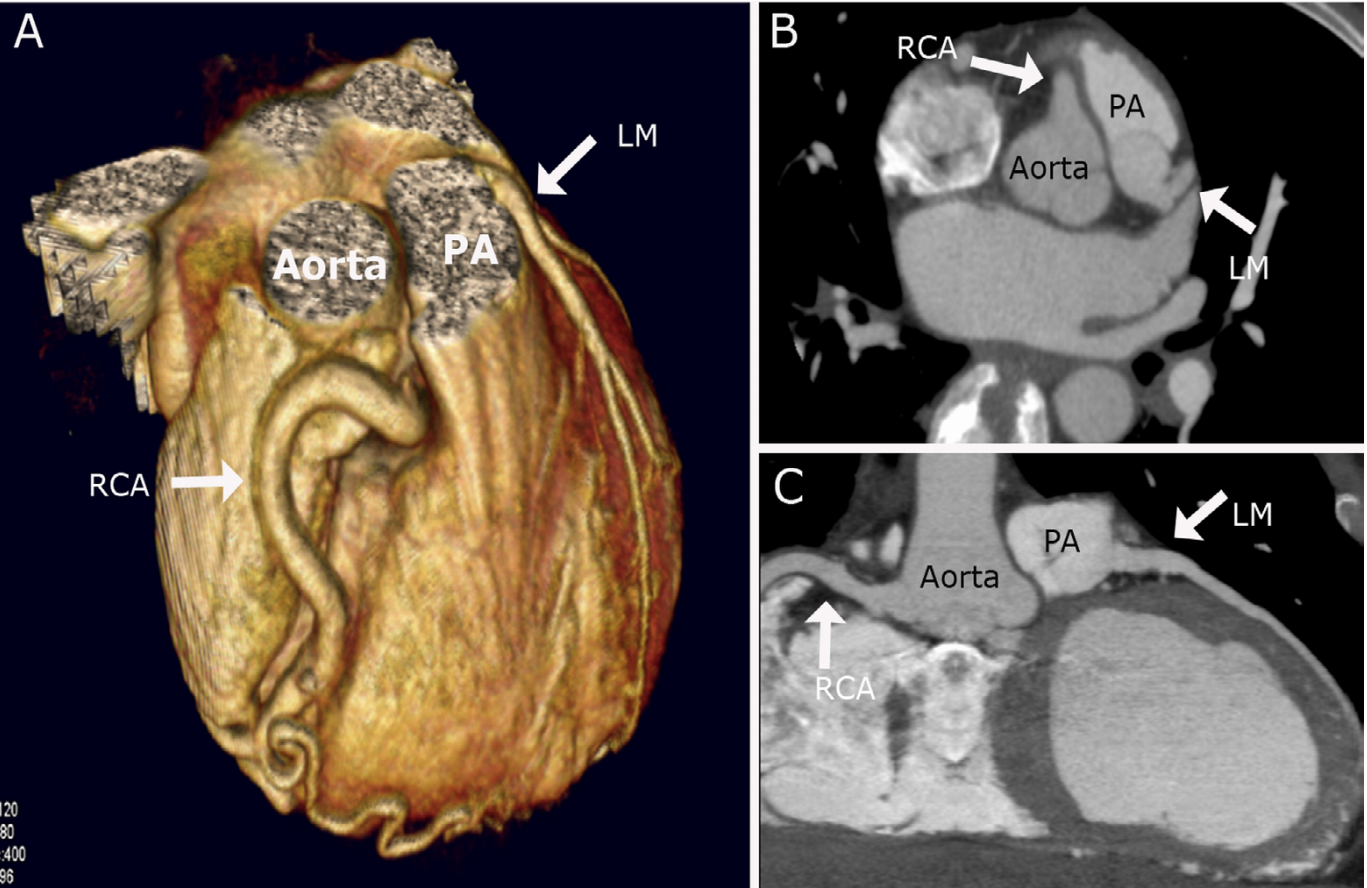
CT-angiography before operation. Coronary CT-angiography showing an anomalous origin of the left main coronary artery (LM) from the pulmonary artery (PA). Normal course of a very large right coronary artery (RCA) is seen. Volume-rendered reformation (A), axial (B) and curved multiplanar reconstruction (C).
